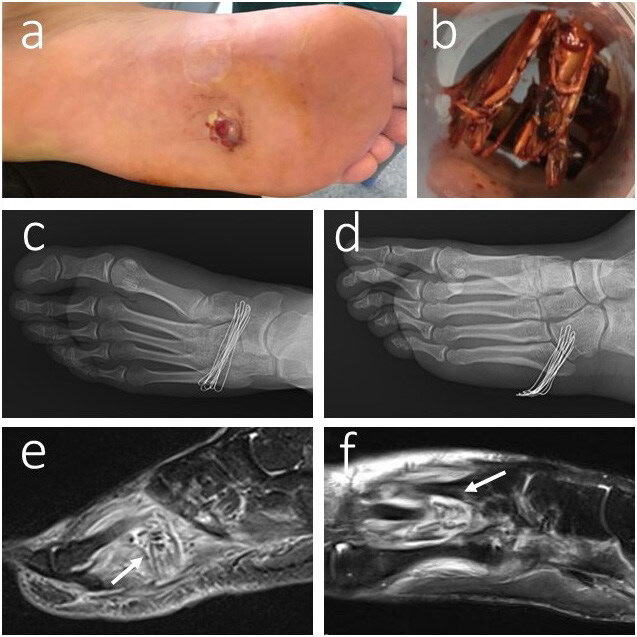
20-year-old male with a wood injury on his left foot. He was admitted 1 week earlier to another hospital and discharged with oral antibiotic therapy only. On his admission to our hospital, there was a discharge, redness, and swelling on his left foot (a); the radiographic image showed nothing related to the FB (c,d); C-reactive protein was 5.23 Mg/L, leucocyte 11.13 × 10^3/μL, and the erythrocyte sedimentation rate were 16 mm/h. Because of this, we decided to get an MRI of the patient, and the MRI-T2 showed abscess formation around the FB (axial view (e) and sagittal view (f)); the wood pieces (diameter range: 4 mm–70 mm) were removed from his left foot (b).
